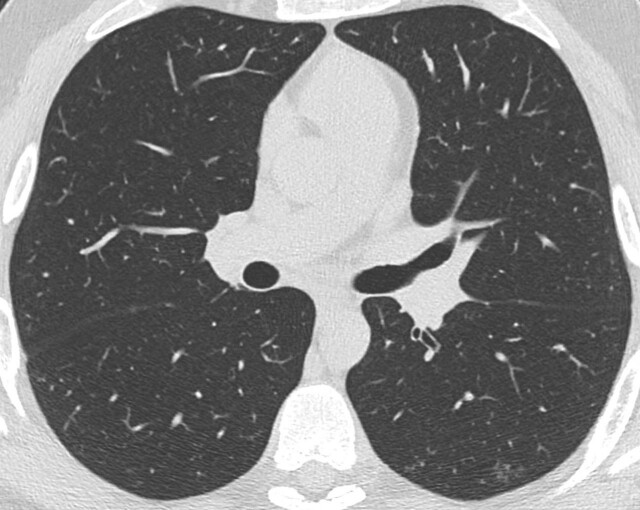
Thin slice (1 mm) lung CT of patients.
